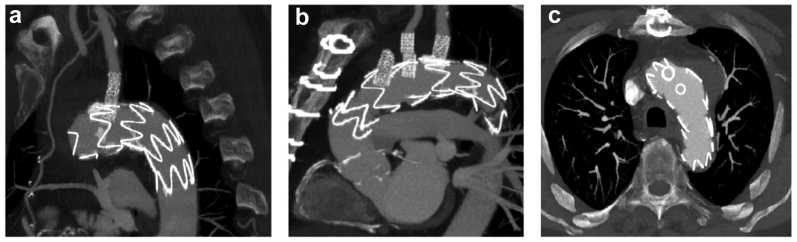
One month follow-up computed tomography scan showing a patent left internal mammary artery (LIMA) to left anterior descending artery (LAD) coronary bypass (*arrow*; a), a patent right coronary artery (b), and the excluded aneurysmal sac with no remaining visible endoleak (c).
